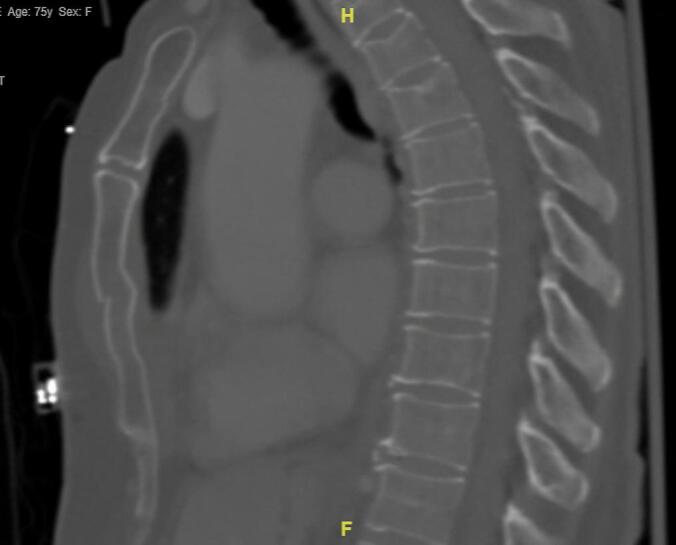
Preoperative CT scan demonstrating displaced sternal fracture in Patient 1.
